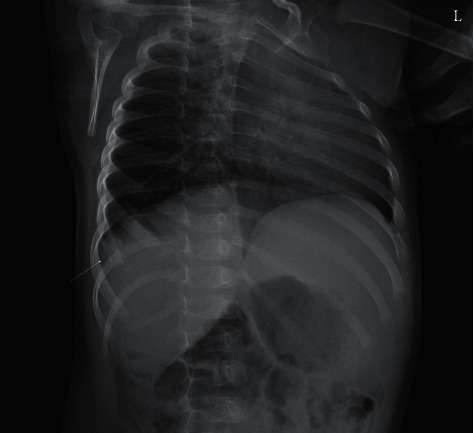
Chest radiograph demonstrated moth-eaten osteolytic lesion at the right anterior 8^th^ rib.
